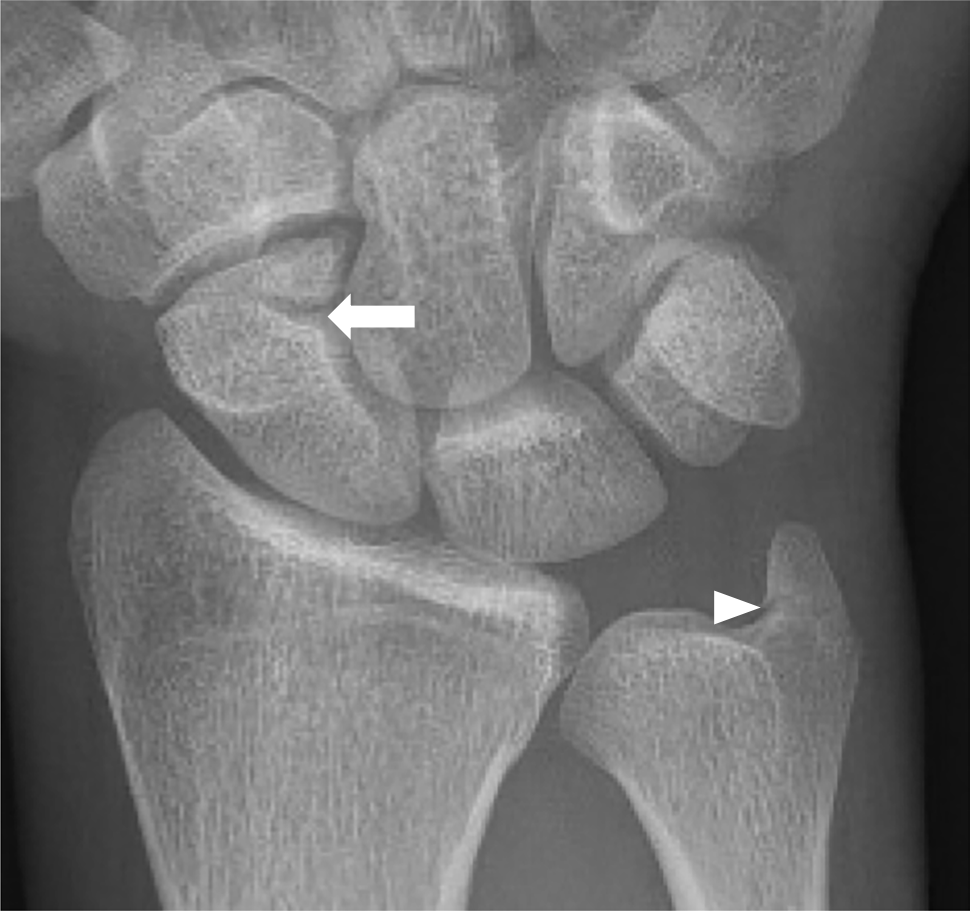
Radiography reveals a fracture of the base of the ulnar styloid (arrowhead), and a line at the distal pole of the scaphoid suggesting a fracture (arrow)
